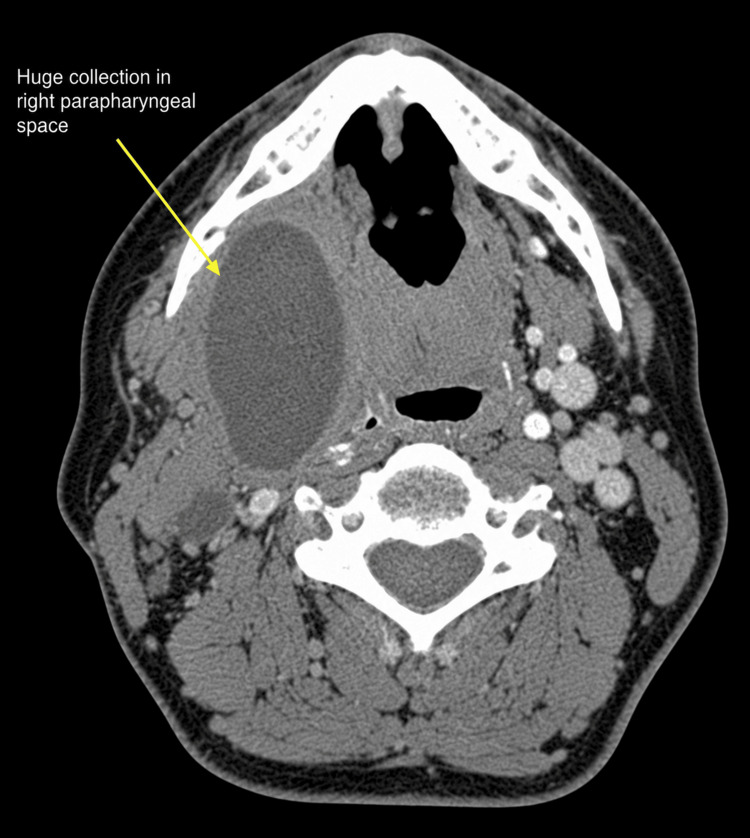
Contrast-enhanced CT scan of the neck showing parapharyngeal space abscess
